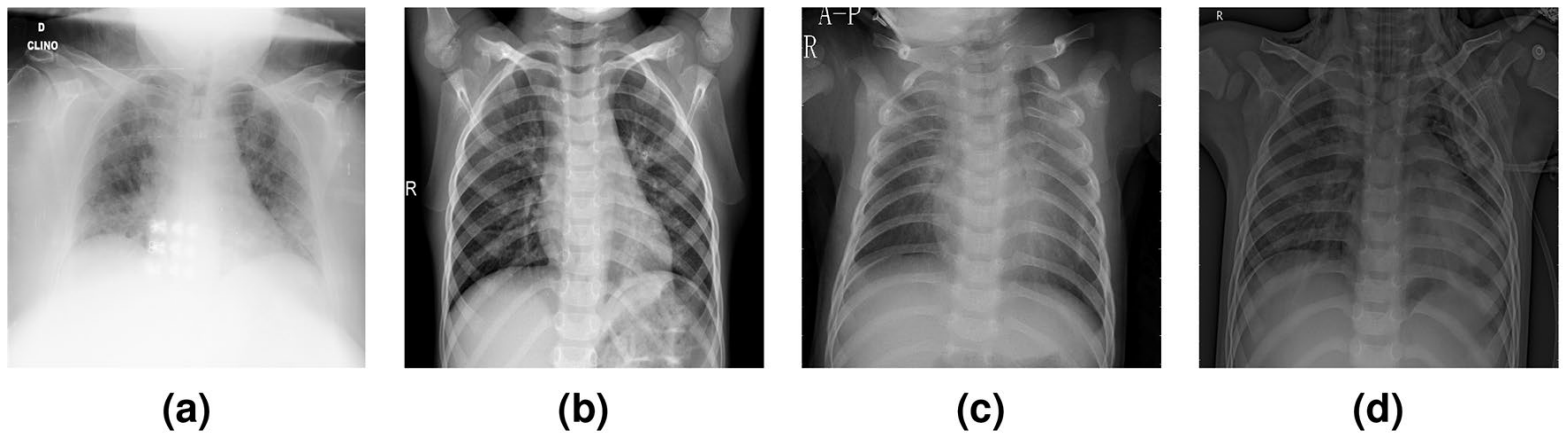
Sample images of chest X-ray images abstracted from CD4^21,23^ for four classes: (a) COVID, (b) Normal, (c) PneumoniaB, and (d) PneumoniaV.
